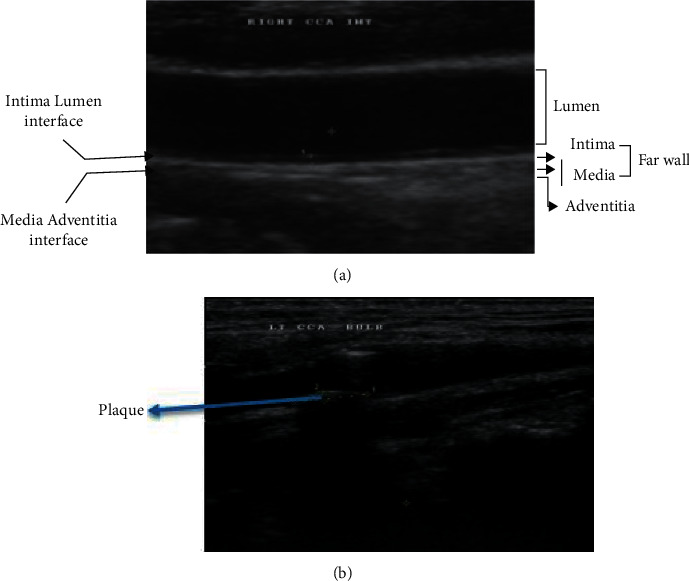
Sample carotid artery ultrasound image (a) with plaque and (b) without plaque.
